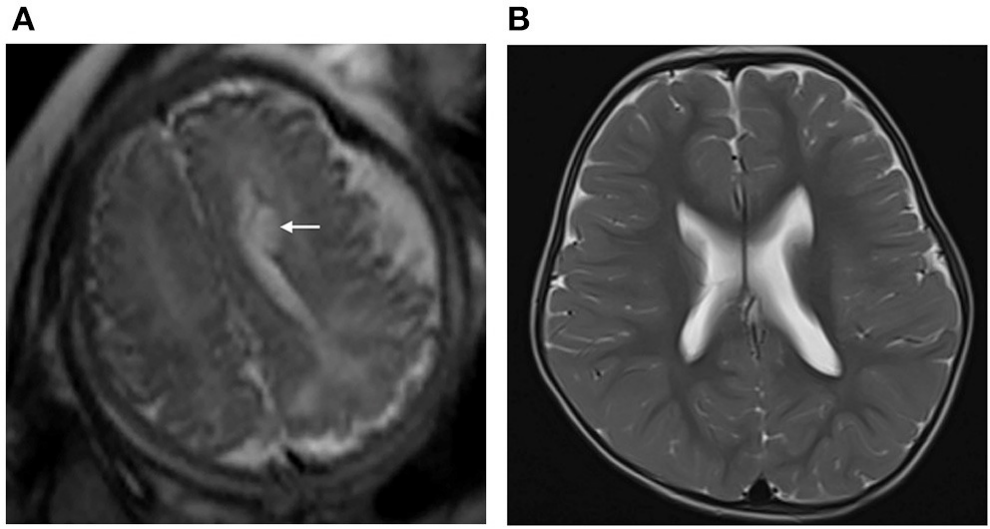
(A) Pregnant woman, 22 years old, with a fetus of 36 weeks' gestation. Fetal brain MRI shows a unilateral isolated periventricular pseudocyst (PVPC) located at the external angle of the lateral ventricle (white arrow); the anteroposterior diameter and height are 0.9 and 0.5 cm, respectively. (B) Female infant, with a history of hypoxic-ischemic encephalopathy (HIE) and asphyxia at birth. At the age of 30 months, the brain MRI showed the PVPC disappeared, and there were no changes in the thalami and basal ganglia related to the previous HIE. The GDS test showed a moderate neurodevelopmental disability in the Adaptive, Gross Motor, and Fine Motor parameters, and mild neurodevelopmental disability in the Language and Personal-Social parameters (DQ:Adaptive: 46, Gross Motor: 50, Fine Motor: 40, Language: 63, and Personal Social: 62).
